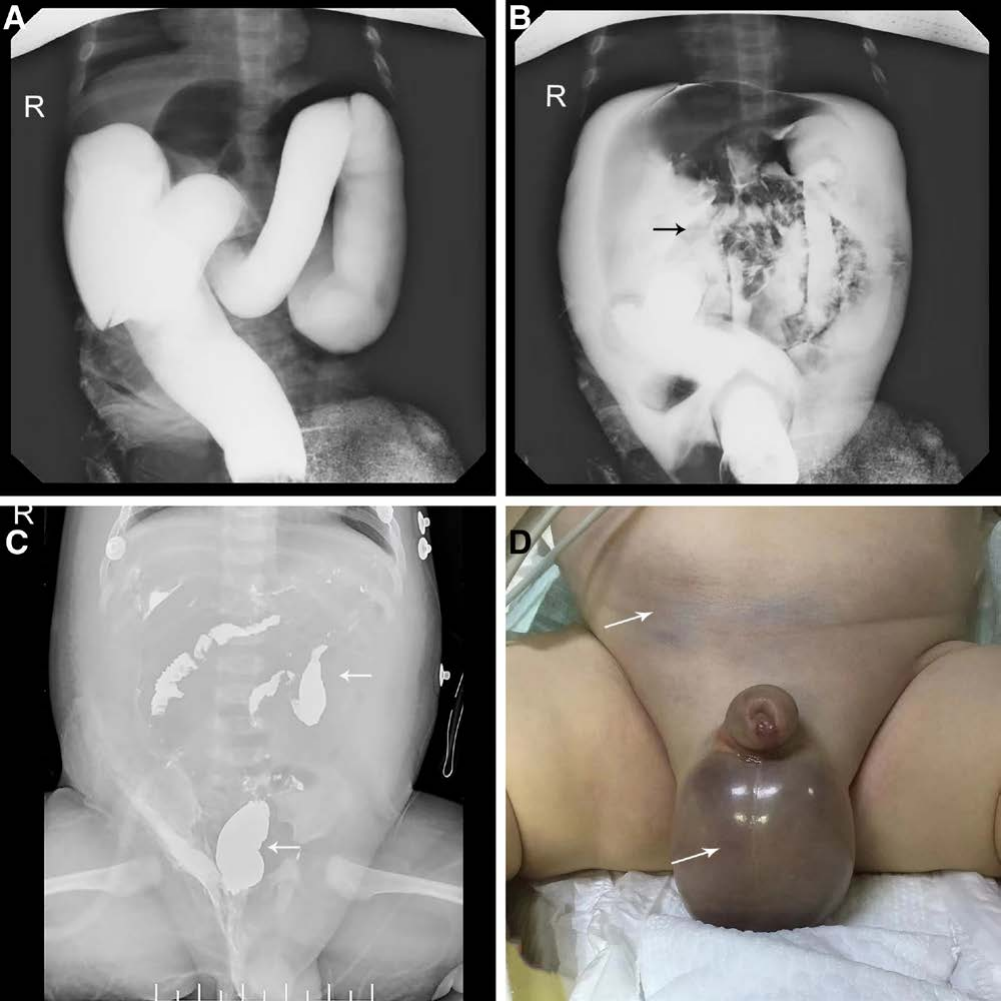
Preoperative abdominal radiographs (A and B). Postoperative abdominal radiographs (C). Preoperative physical manifestations (D).
